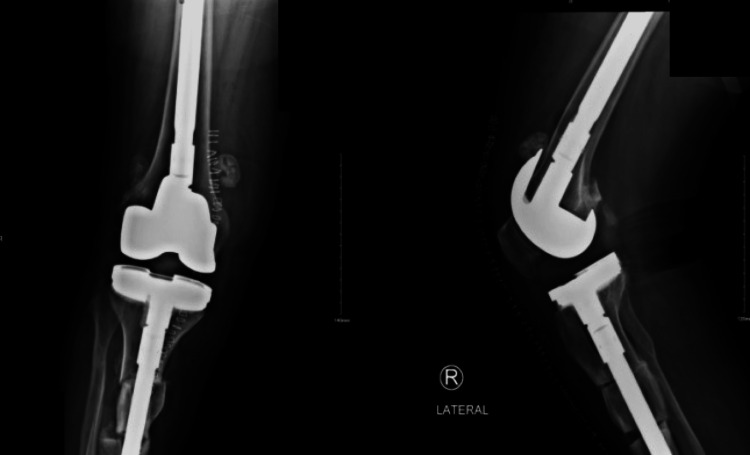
Right side anterior-posterior and lateral view radiograph for total knee arthroplasty
